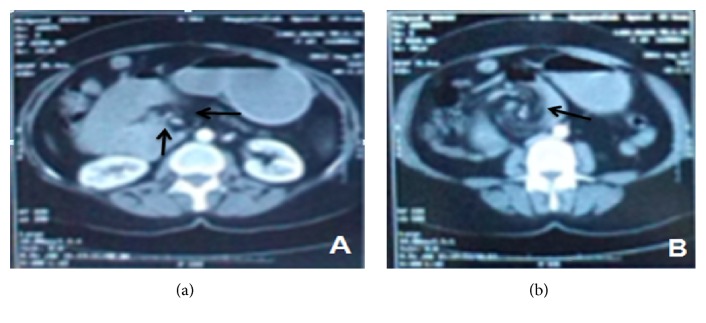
(a-b) CT scans obtained at the time of hospital admission. (a) shows collaterals of superior mesenteric veins surrounding the superior mesenteric artery. In (b) bowel is twisted around central superior mesenteric artery.
